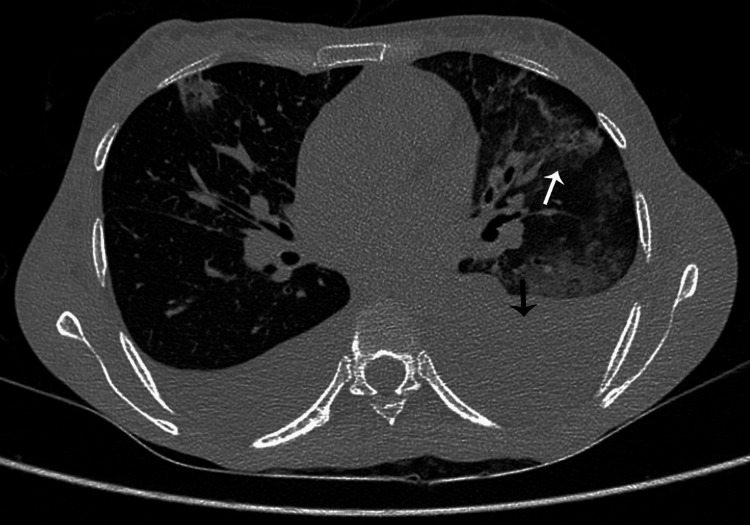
Bilateral pleural effusion (black arrow) and pulmonary edema (white arrow) noted in the HRCT of thorax
